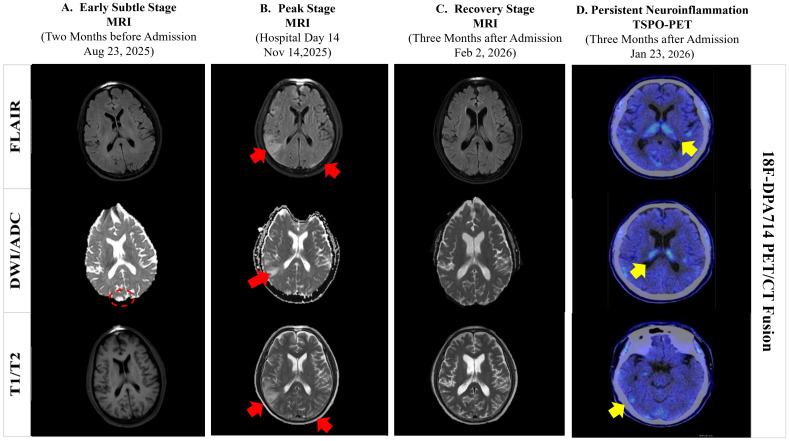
Multistage neuroimaging evolution in anti-GABA_A_ receptor encephalitis. Representative structural and molecular neuroimaging findings across different stages of the disease course. (A) Early stage (Two Months before Admission, Aug 23, 2025). Brain MRI shows a subtle focal cortical-subcortical signal abnormality in the right parieto-occipital region with mild hyperintensity on T2-FLAIR without diffusion restriction or contrast enhancement. The dashed circle highlights this small and nonspecific lesion. (B) Peak disease stage (Hospital Day 14, Nov 14, 2025). Brain MRI demonstrates extensive bilateral cortical-subcortical hyperintensities on T2-FLAIR and T2-weighted sequences, consistent with diffuse inflammatory involvement in AE. Red arrows indicate representative areas of cortical-subcortical signal abnormality. (C) Recovery stage (Three Months after Admission, Feb 2, 2026). Follow-up MRI shows near-complete resolution of the previously observed abnormalities. (D) Persistent neuroinflammation on TSPO-PET (Three months after admission). 18F-DPA714 PET/CT fusion images demonstrate persistent increased tracer uptake in the bilateral temporo-parieto-occipital cortices despite substantial structural recovery on MRI. Yellow arrows indicate representative regions of increased TSPO tracer uptake, consistent with persistent neuroinflammatory activity.
